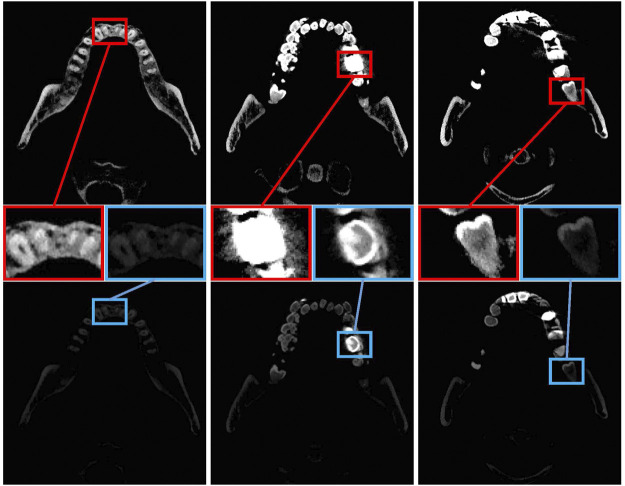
Tooth CT images of three slices. The top and bottom lines represent the CBCT images of teeth obtained by setting different window widths and window positions. Teeth or metal may not be separated when the window width is not set correctly. The top line set the window width and window level to 3,010 and 2,006. In the bottom line, the window width and window level are set to 3,074 and 4,202, respectively, as confirmed by the expert.
